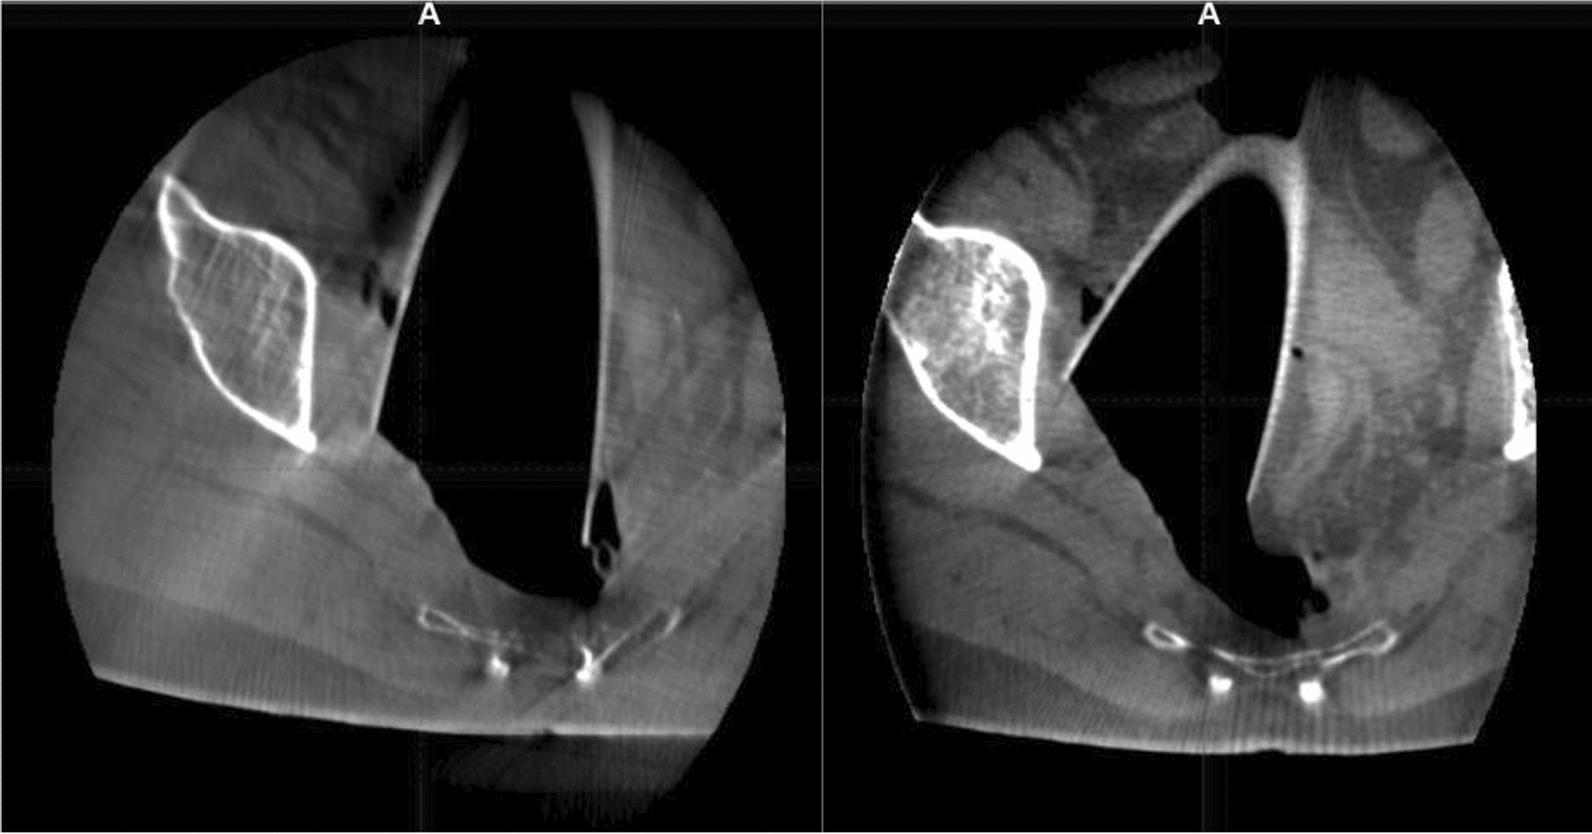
Cone-beam CT scans prior to and after correction of the applicator position
